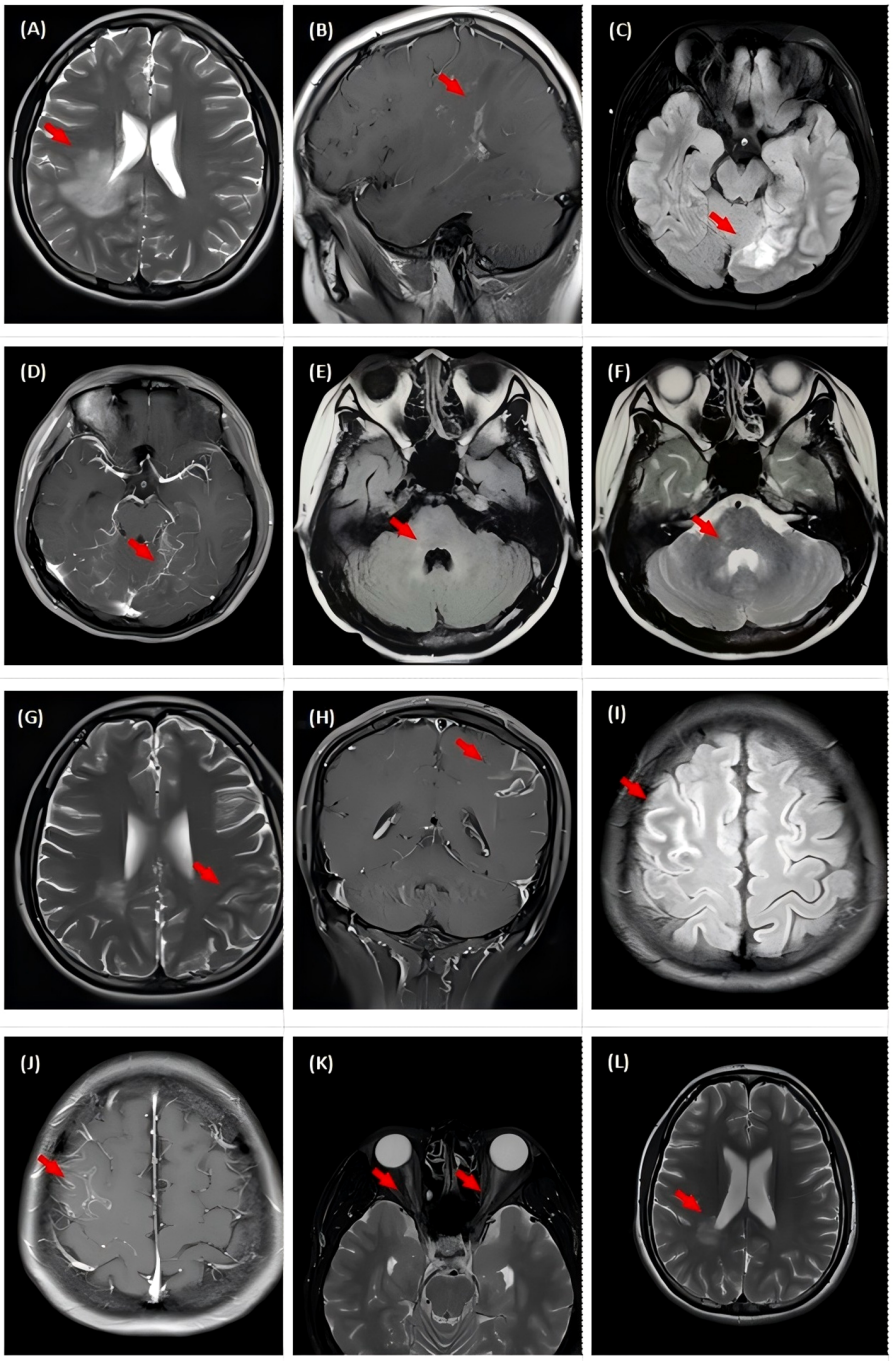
Magnetic Resonance Imaging of the brain and spinal cord. (A, B) Brain MRI at onset showing abnormal signals on the right corona radiata and basal ganglia regions. (C, D) Brain MRI showing alterations on left temporal an occipital lobes with enhancement on FLAIR and T1 post-Gd during second attack. (E, F) Abnormal signal on T2/FLAIR in the right pons at the time of the patient’s third attack. (G, H) New lesion in the left parietal lobe with contrast-enhancement during fourth attack. (I, J) New lesions in the right frontal cortex with linear contrast-enhancement during fifth attack. (K) Abnormal signal in both optic nerves, following treatment with RTX at the time of the patient’s sixth attack. (L) Lesions after 1 year of treatment with telitacicept.
